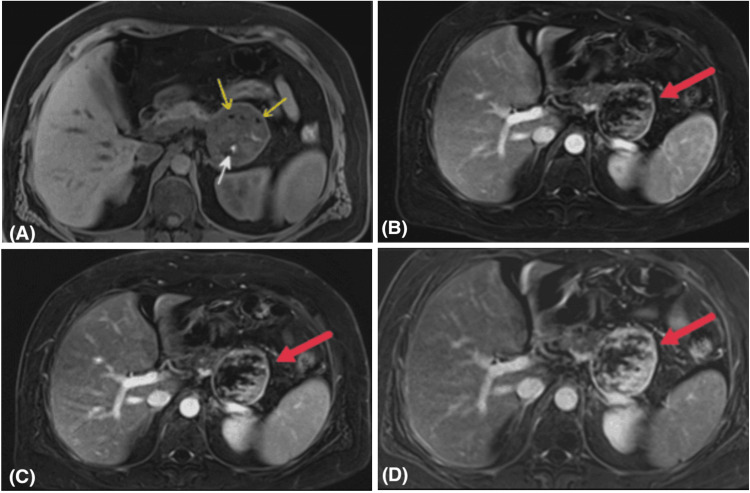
MRI of the abdomen: axial plane T1 fat saturation (sat) (A), arterial phase T1 fat sat (B), venous phase T1 fat sat (C), and delayed phase T1 fat sat (D) images showing the well-defined mass arising from the pancreatic tail with fat foci (yellow arrows), hemorrhagic foci (white arrow) and progressive heterogeneous enhancement (red arrows).
